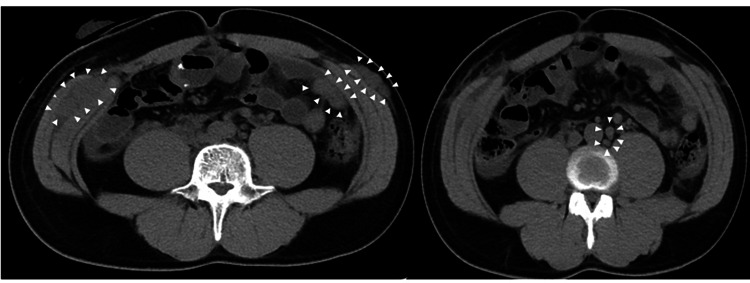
Multiple metastases after the surgery. A computed tomography scan performed four months after the surgery exhibits multiple metastases involving the abdominal wall and para-aortic lymph nodes (white arrowhead).
